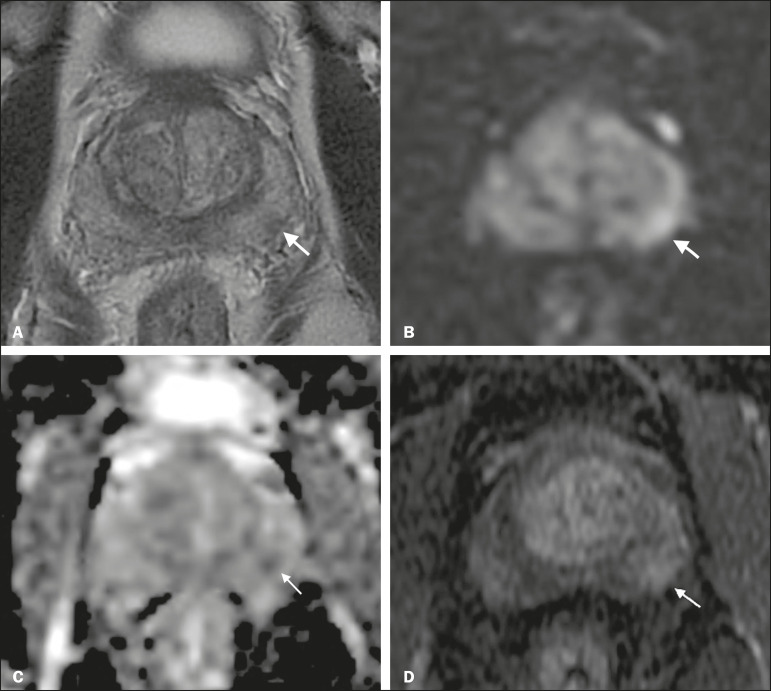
A 62-year-old patient with PCa classified as International Society of Urological Pathology (ISUP) grade 1 (Gleason score of 6) in 2 of 12 cores in a systematic biopsy. After he had been in an AS protocol for one year, mpMRI was requested. A: Axial T2-weighted image showed a small, ill-defined hypointense area. B,C: DWI (b = 1400 mm/s^2^) and ADC map showing a small focus of restriction at the same location identified on the T2-weighted image. D: Dynamic contrast-enhanced imaging showing early enhancement of the same area. The patient was withdrawn from AS, operated on and an ISUP 3 (Gleason score of 4+3) was found at surgery.
